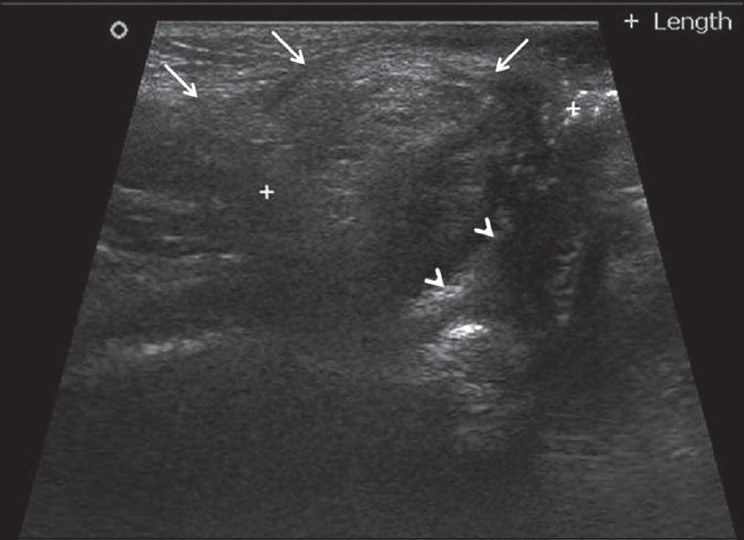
Ultrasound of the perineum shows the mass (arrows) posterior to the rectum (arrow heads)
